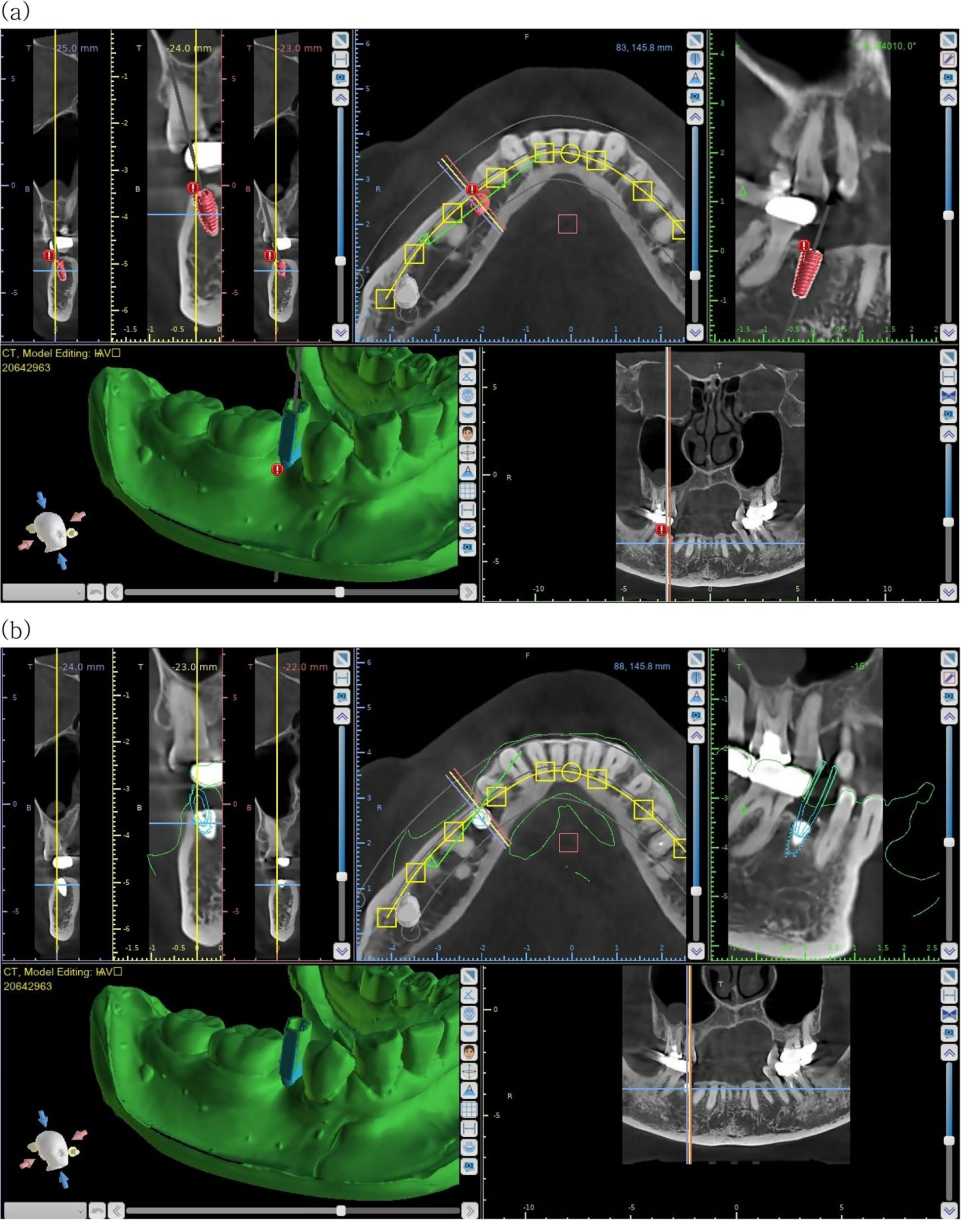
a Overlay fixture combined with the scan abutment on the cast with the planned implant in the Blue Sky Plan III software and extracted as an STL file. b The implant fixture is superimposed on the postoperative CBCT in the Blue Sky Plan III software and extracted as an STL file
